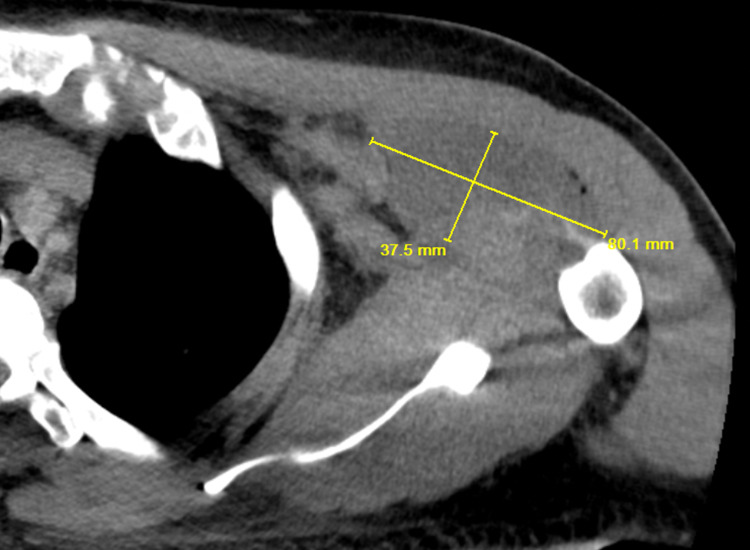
CT of left shoulder joint showing a hypodense collection (yellow lines showing dimensions, length and breadth of the collection) with multiple foci of air concerning for intramuscular hematoma/abscess which measures 8.0 x 3.8 cm in the anterior left shoulder, specifically the musculature of the anterior deltoid and pectoralis major/minor.
